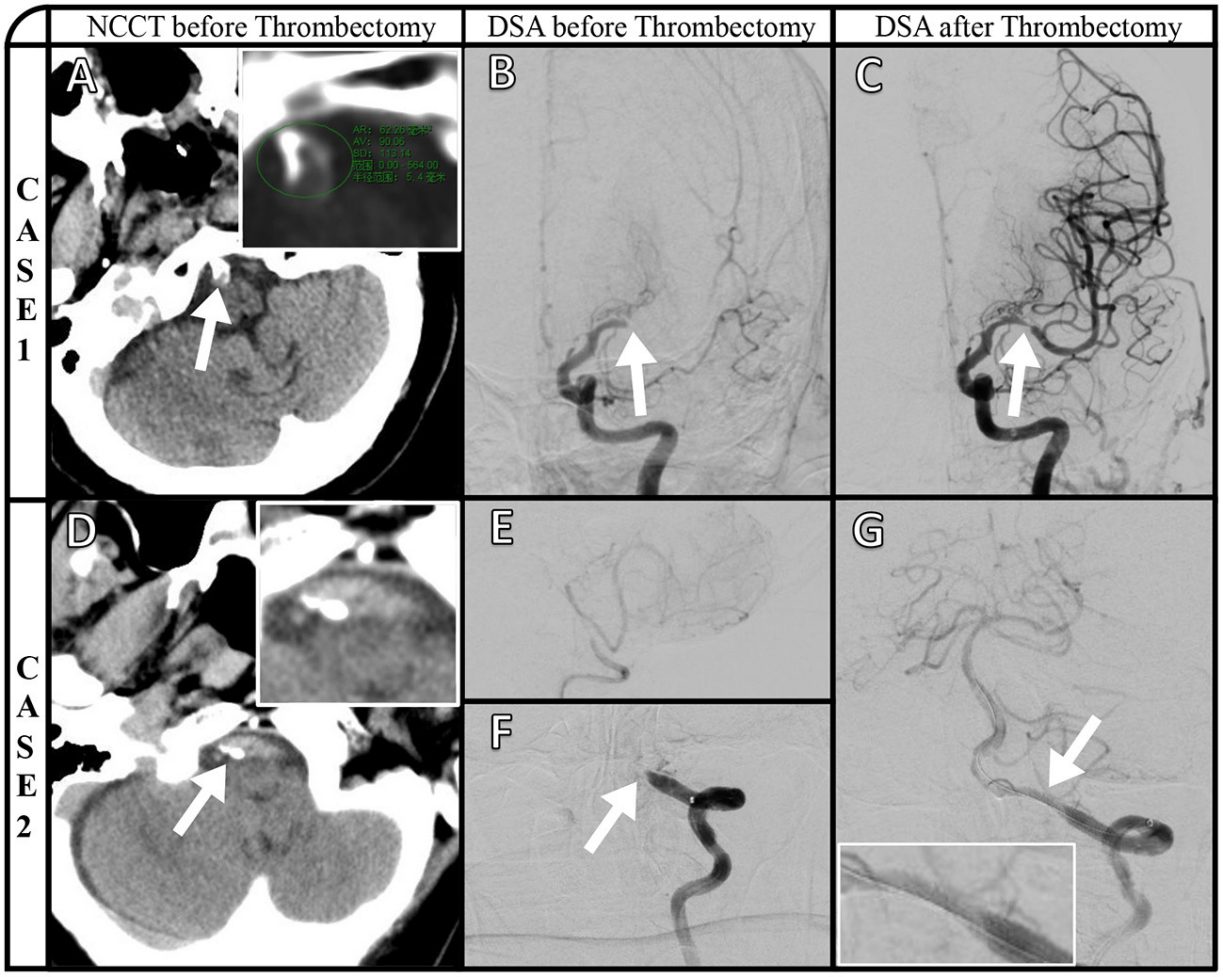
Representative images of VBAC and ICAS-O in thrombectomy (CASE1 and CASE2). Axial NCCT image (A) and magnified view (insert in A) show basilar artery calcification, and the highest CT value is 564HU. Posteroanterior left internal carotid artery angiography (B) manifested left middle cerebral artery occlusion. The stenosis (C) after thrombectomy demonstrates the etiology is ICAS occlusion. Axial NCCT imaging from a different patient (D) and magnified view (inset in D) show left vertebral artery calcification. Right vertebral artery angiography (E) shows that only supply posterior inferior cerebellar artery, and posteroanterior left artery angiography shows (F) V4 occlusion. The stent (G) and magnified view (insert in G) released *in situ* ICAS occlusion.
